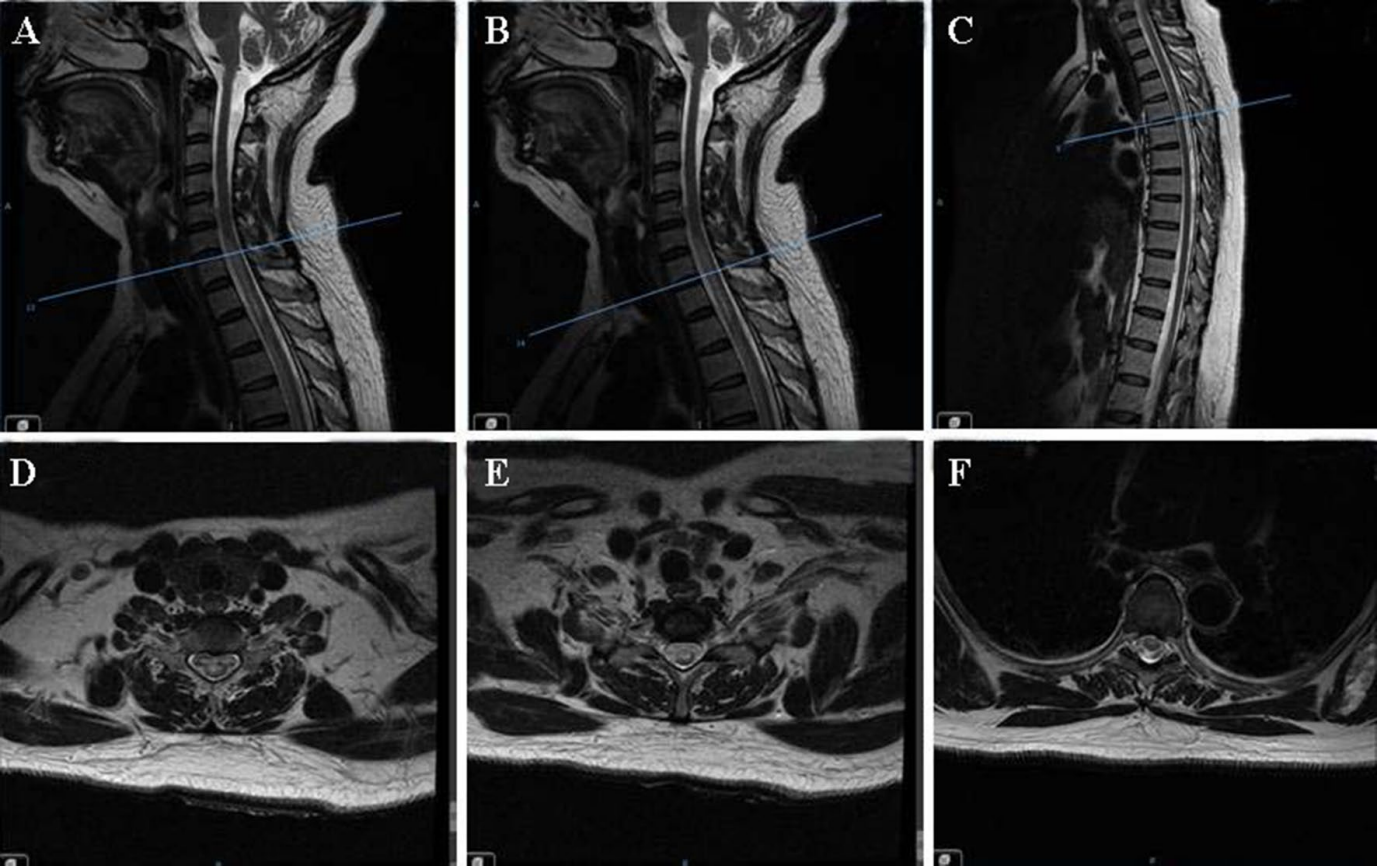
Magnetic resonance imaging (MRI) obtained on the third day of onset of the disease (sagittal section A–C; transverse section corresponding to the scaleplate respectively D–F). T2 hyperintense linear mass best appreciated on sagittal sections, showing long segment myelitis, extending from C5–T2
